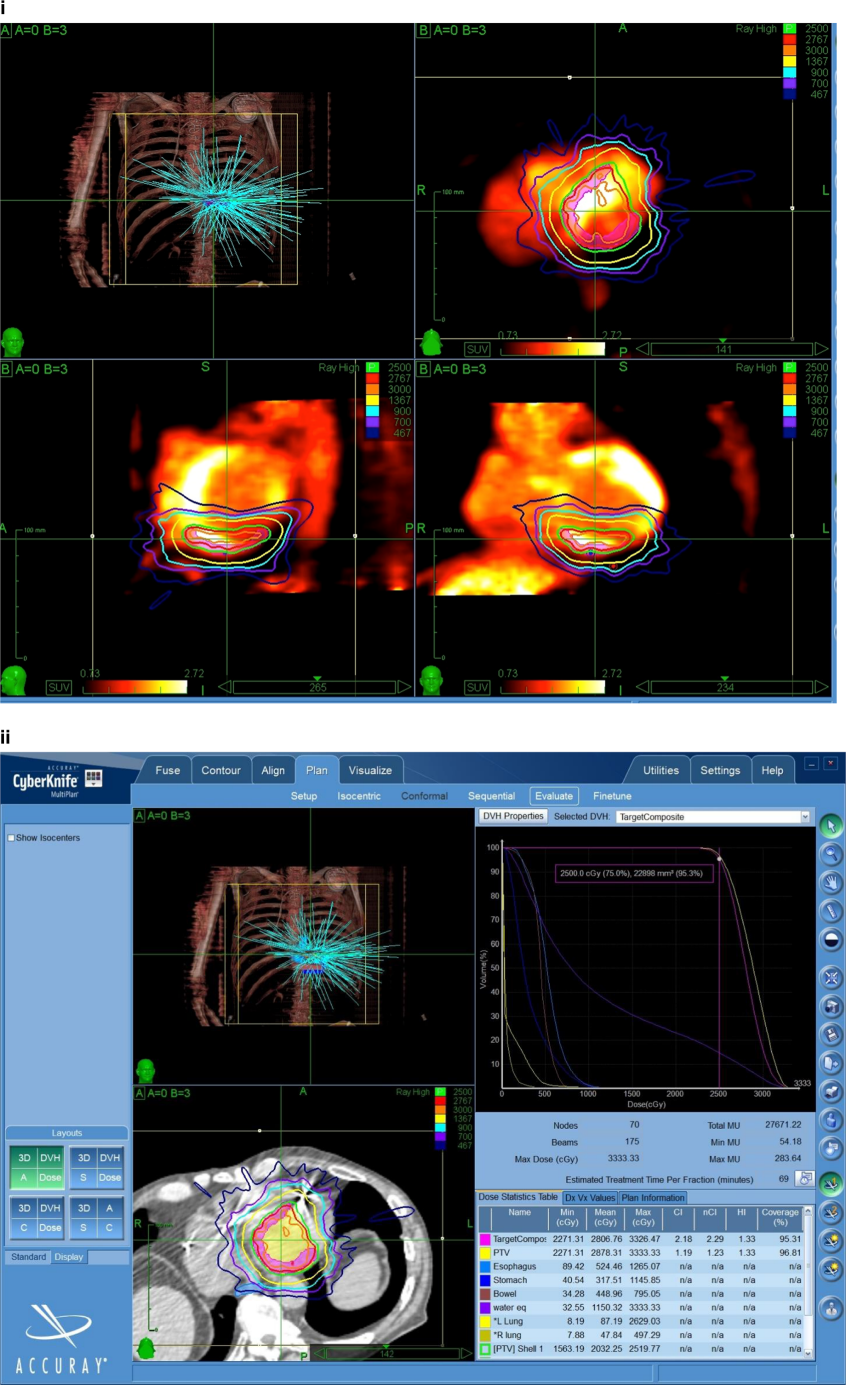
Treatment of a scar-based VT is shown. In part *i*, PET imaging demonstrating dense infarct in the inferobasal wall of the left ventricle (LV) is shown. The intended treatment plan is superimposed, along with planned delivery beams in three dimensions, as well as planned isodoses. In part (*ii*), the treatment software display is shown, with CT imaging of the intended inferobasal LV target shown. Superimposed on the CT image are delivered radiation beams and delivered isodoses
